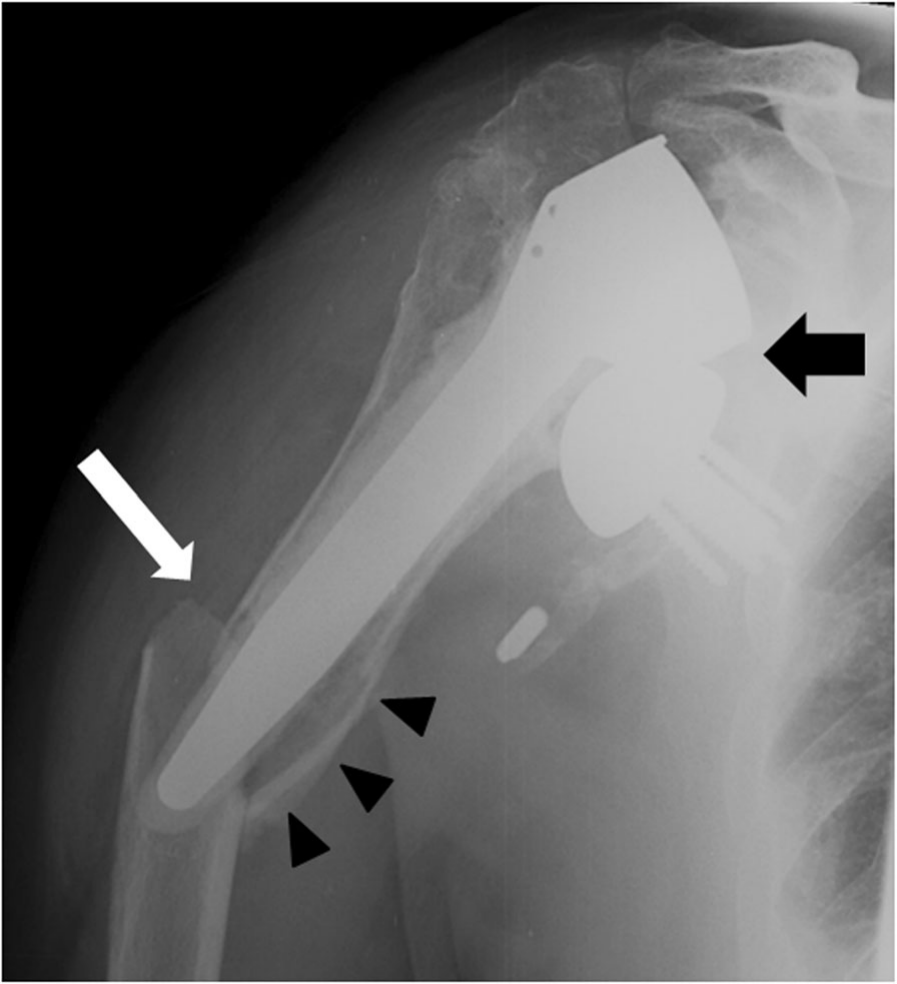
Anteroposterior radiograph of a 73-year-old man shows a periprosthetic fracture (white arrow) and a complex glenohumeral dislocation (black arrow). Note that there was previously a stress shielding manifested by cortical thinning and radiolucency area around the distal humeral stem (arrowheads)
